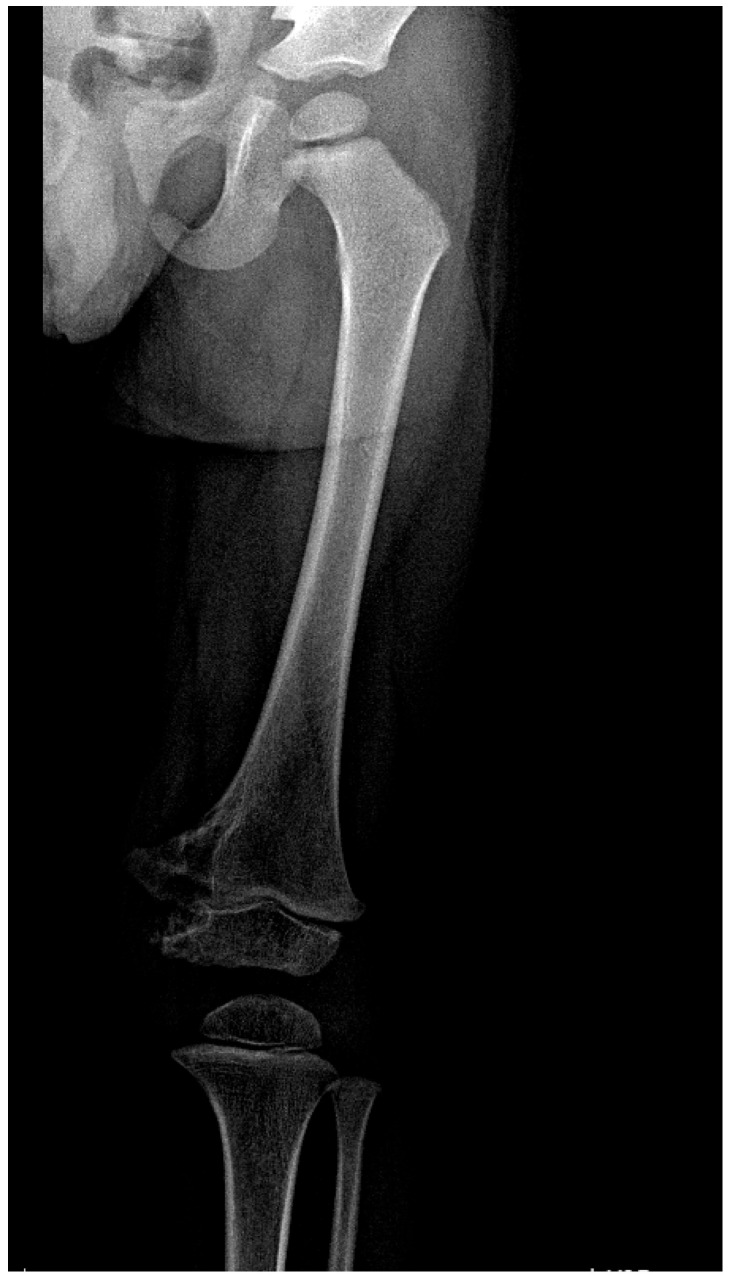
X-ray of the left knee reveals mixed osteocondensing and osteolytic regions in the distal third of the left femur, with the coxo-femoral joint remaining intact.
